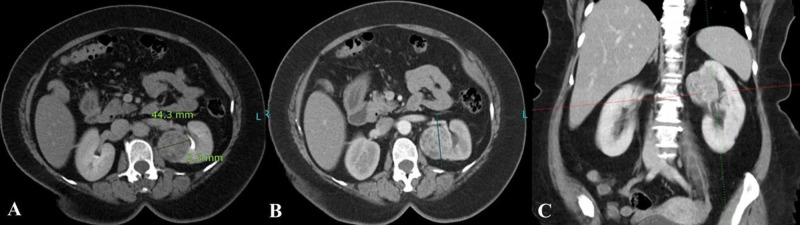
Moderate complexity lesion (8p) according to the RENAL nephrometry score A) Excretory phase of the triphasic CT scan showing a left renal lesion with a diameter of 4.4 cm, which is 2 mm away from the renal collecting system. (B) Venous phase contrast-enhanced CT scan showing > 50% of the lesion being exophytic. (C) Coronal reformatted image showing the lesion crossing the polar line. RENAL: radius, exophytic/endophytic properties, nearness of tumor to the collecting system or sinus in millimeters, anterior/posterior, location relative to polar lines
